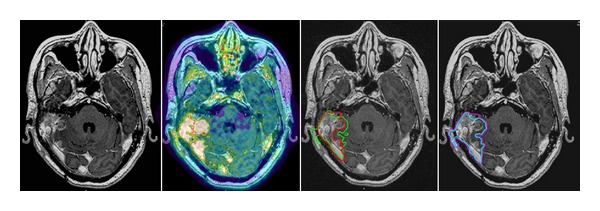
A 50-year-old male patient (patient no. 13) with a recurrent squamous cell cancer of the right petrous bone. The extent of infiltrative intracerebral extension was not delineable to the full extent by MRI. The MRI/CT volume is delineated in red and the PET volume in green. The additional PET information is included in the initial treatment fields (magenta). Note the reduction in the boost volume delineated in turquoise.
